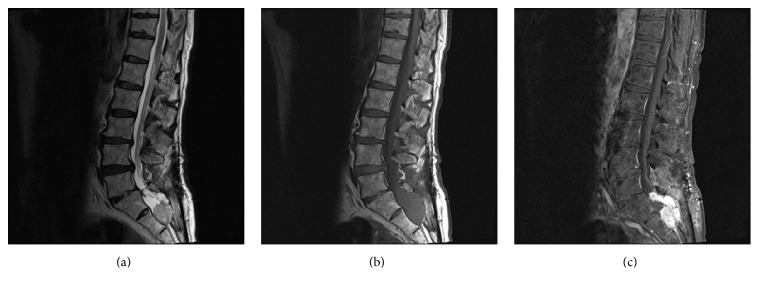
MRI of the lumbar spine with and without contrast. (a) Sagittal T2, (b) sagittal T1, and (c) sagittal T1 after fat saturation: significant enlargement of previously noted sacral mass with new bony erosion and significant mass effect within the spinal canal causing displacement of posterior spinal structures. The mass has taken a more lobulated appearance as seen on the T2 images and is vividly contrast enhancing.
